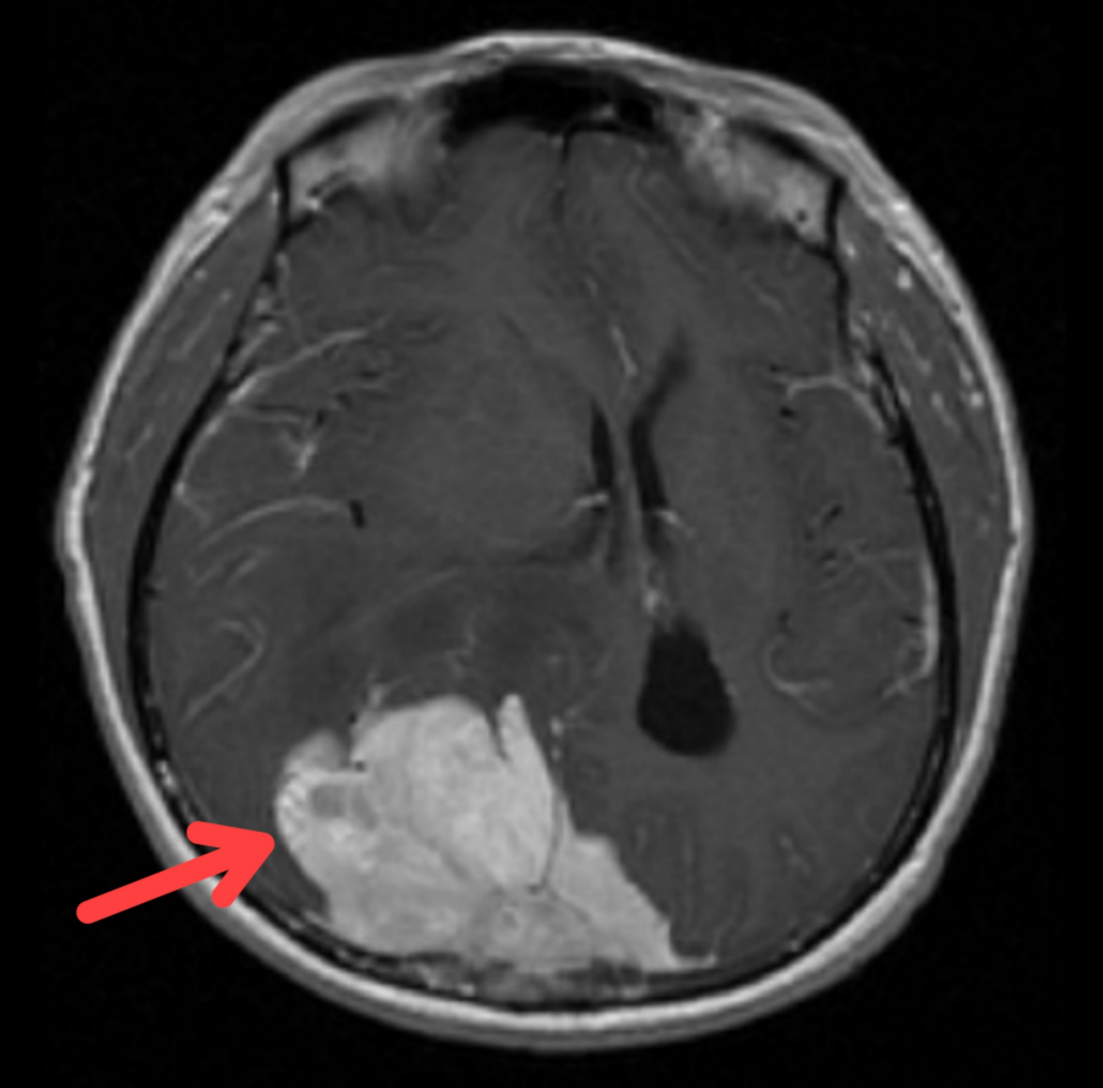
Magnetic resonance imaging showed a huge right occipital lobe tumour with mass effect.
